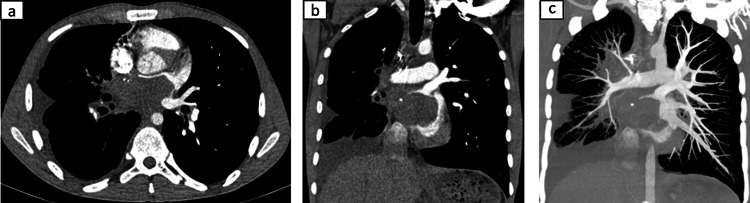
(a) Axial CT. (b) Coronal CT. (c) Coronal CT, MIP (a,b) Contrast-enhanced CT of the chest revealed a large, lobulated, nonenhancing heterogeneous mass occupying nearly the entire left atrial cavity. (c) Complete thrombosis of the right superior and inferior pulmonary veins CT: computed tomography; MIP: maximum intensity projection
